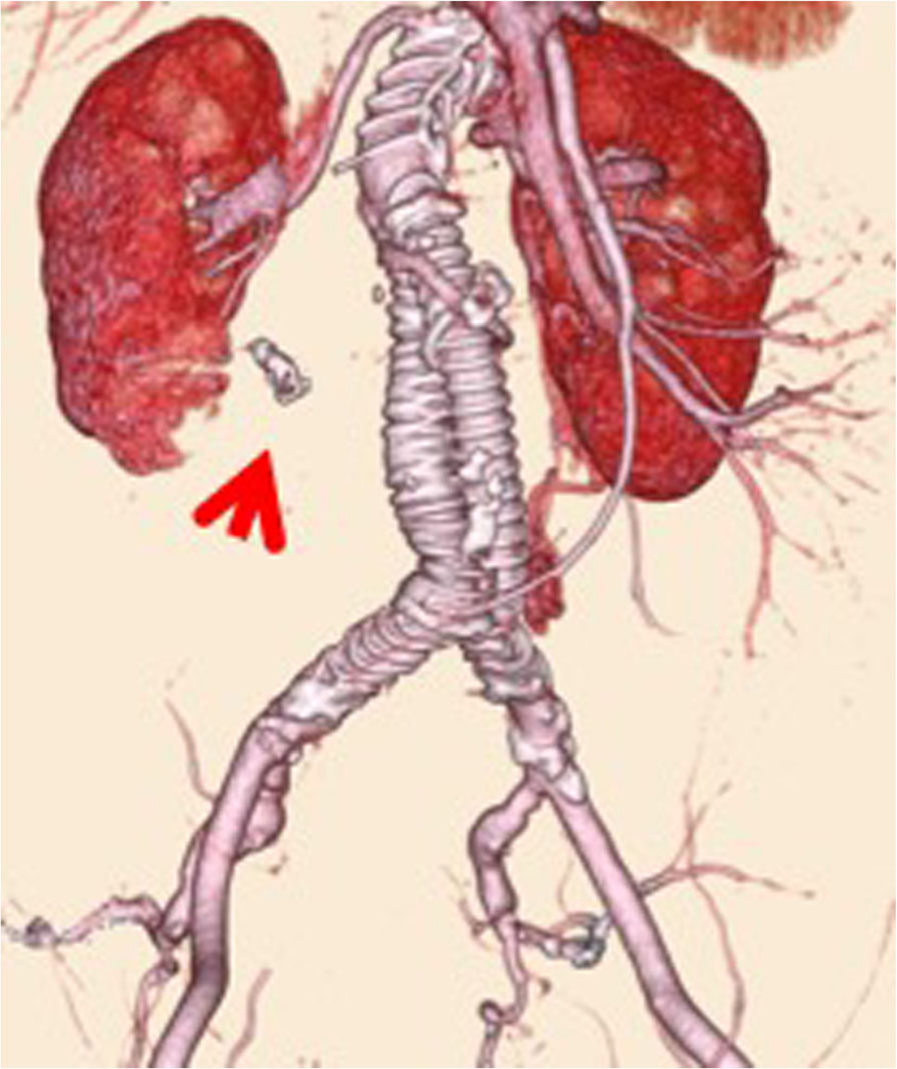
Post-operative enhanced CT. There was no type II endoleak from the ARA. The distal portion of the ARA from the coils was well perfused (*arrow*) and the right kidney was not infarcted
